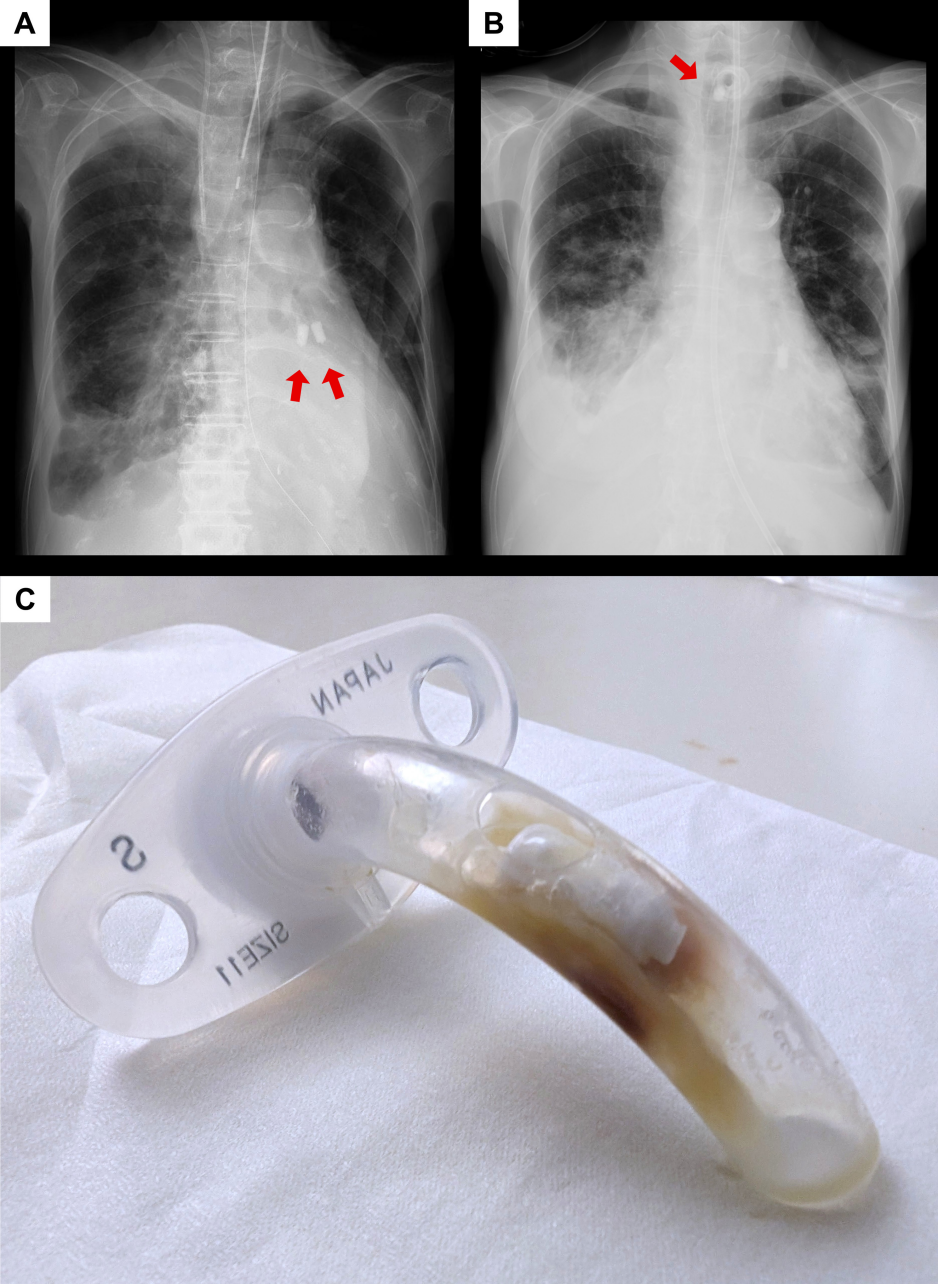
(A) Portable chest radiography (anteroposterior view, supine) demonstrated the initial placement of endobronchial Watanabe spigots in the left lateral basal segmental bronchus (B9) and posterior basal segmental bronchus (B10) (arrows). (B) Chest radiography (posteroanterior view, upright) demonstrated a foreign body (arrow) within the tracheostomy tube. (C) The migrated endobronchial Watanabe spigot (EWS) can be seen obstructing the lumen of the removed tracheostomy tube.
